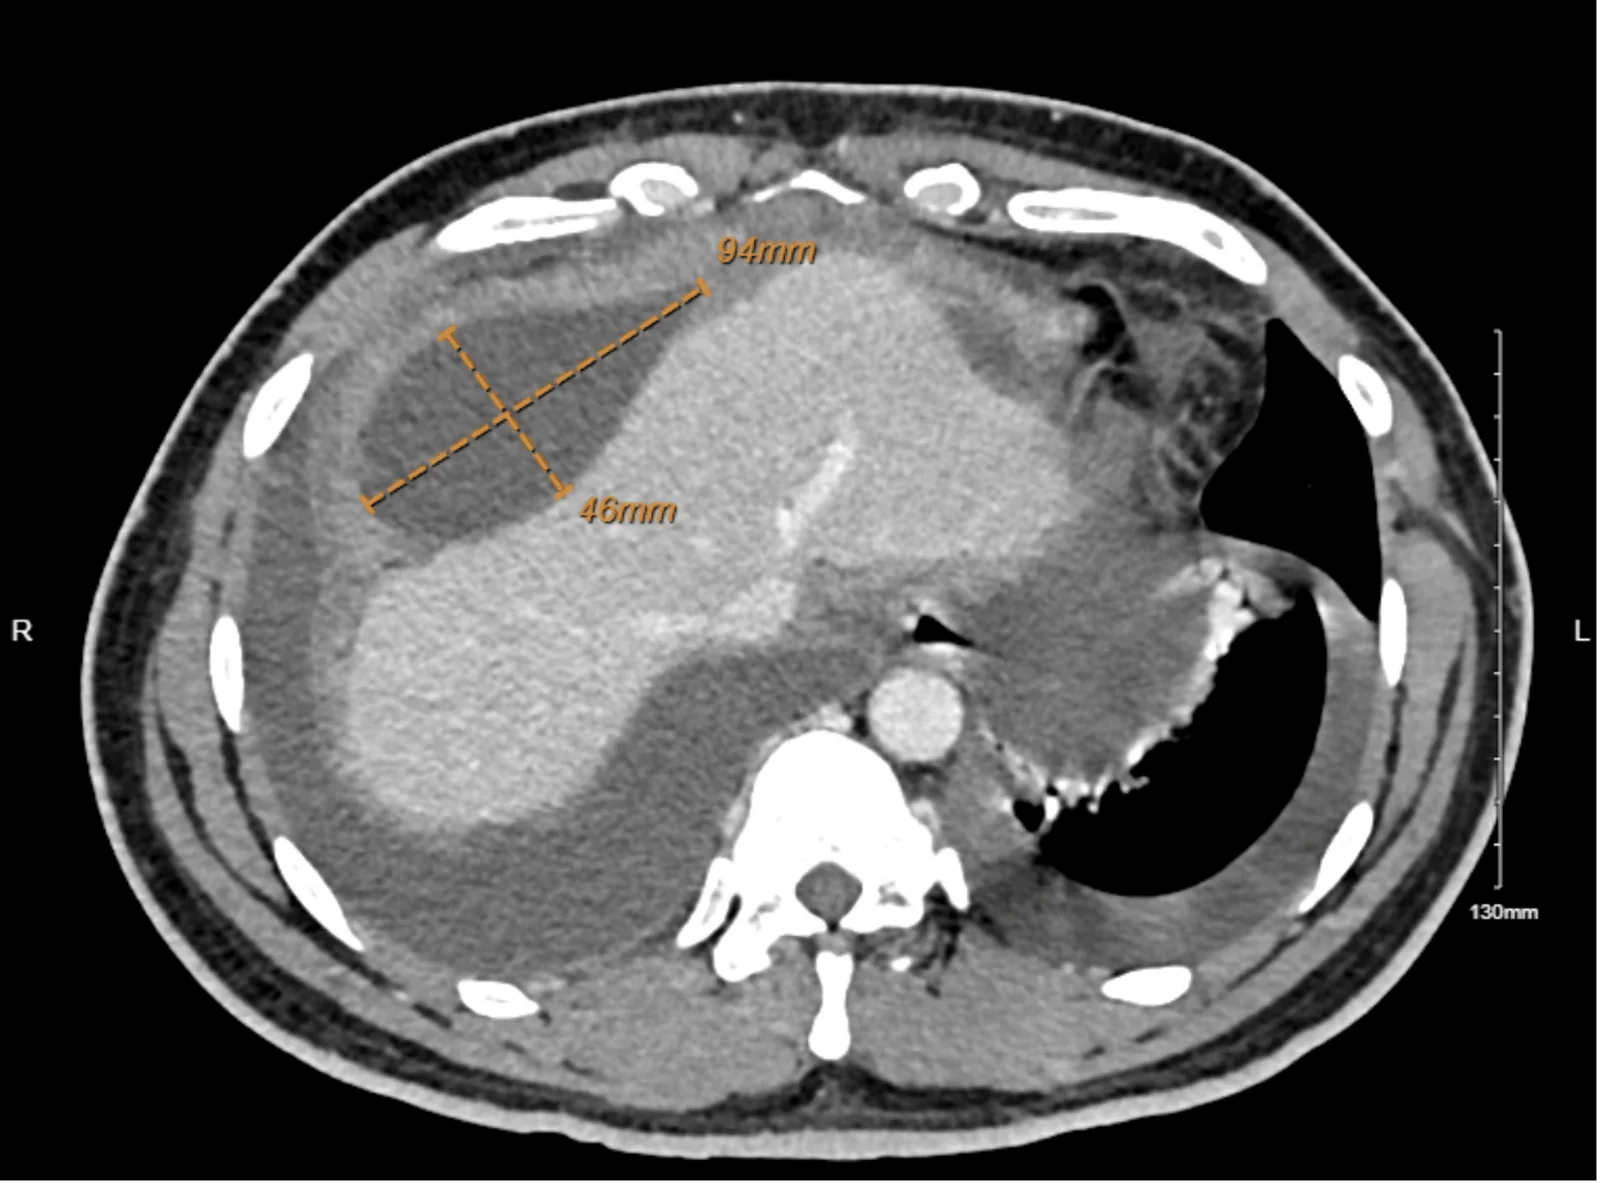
Contrast-Enhanced CT Abdomen and Pelvis Demonstrating Loculated Ascites Contrast-enhanced computed tomography (CT) of the abdomen and pelvis obtained in April 2024 demonstrating loculated ascites throughout the abdomen, including a large perihepatic fluid collection measuring 4.6 × 9.4 × 9.3 cm.
